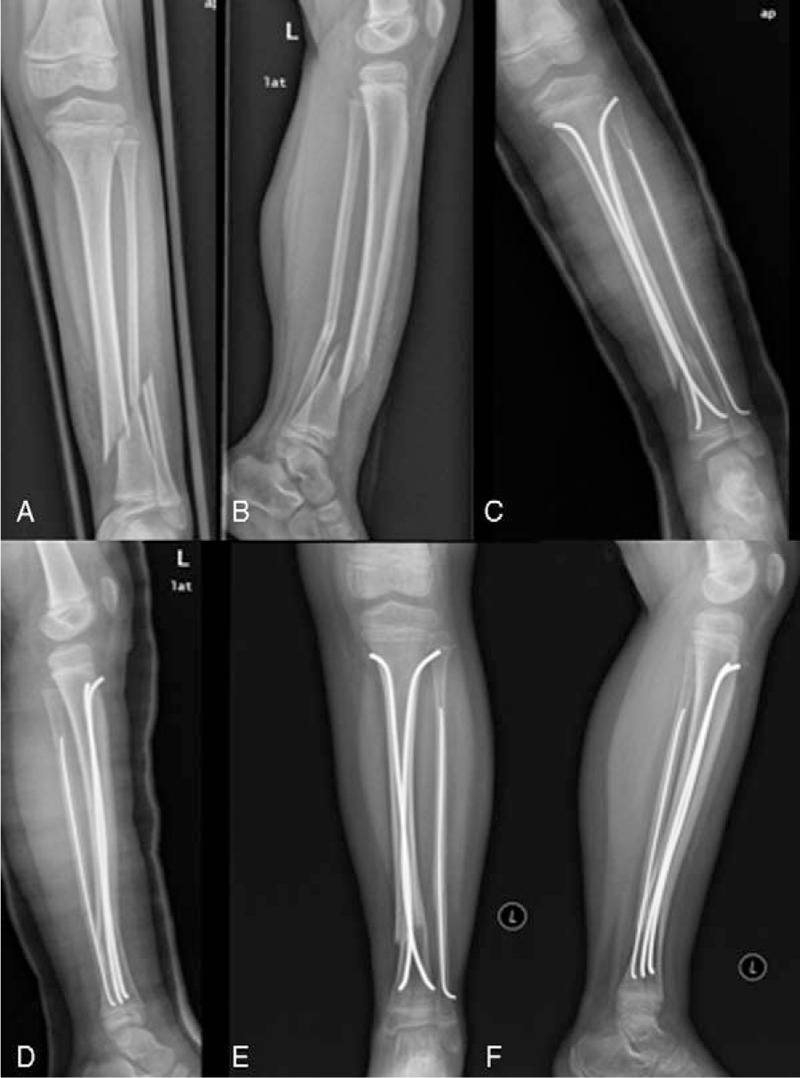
A 7.1-year-old boy suffered traffic accident. A, B, Anteroposterior and lateral radiographs of the left leg with mild oblique unstable DTDMJ fracture. C, D, Immediate postoperative radiographs after closed reduction and fixation with ESINs. E, F, 6 months after surgery, the fracture showed radiographic evidence of healing.
